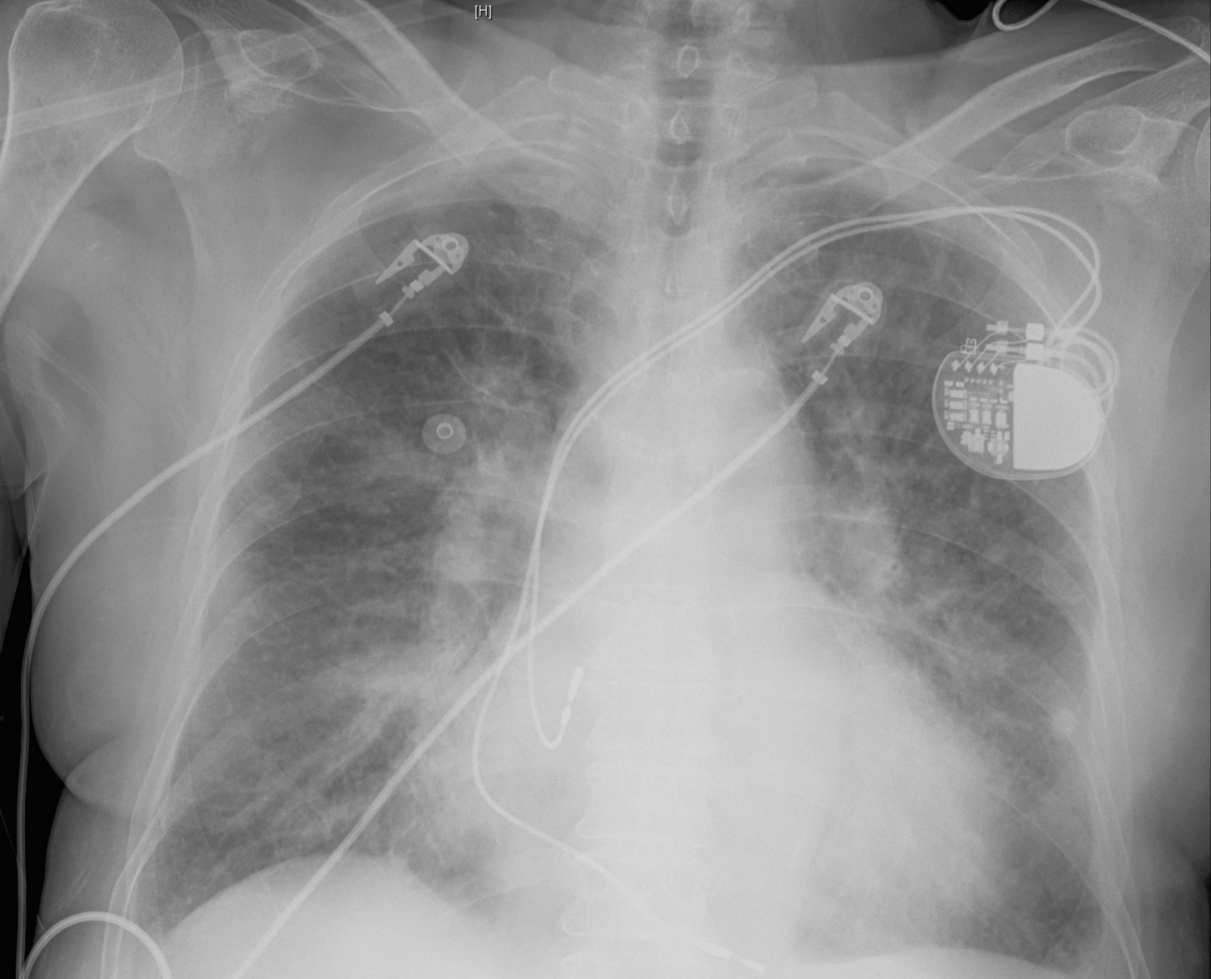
Chest radiograph The radiograph is suspicious for congestive heart failure with pulmonary edema versus multifocal pneumonia.
